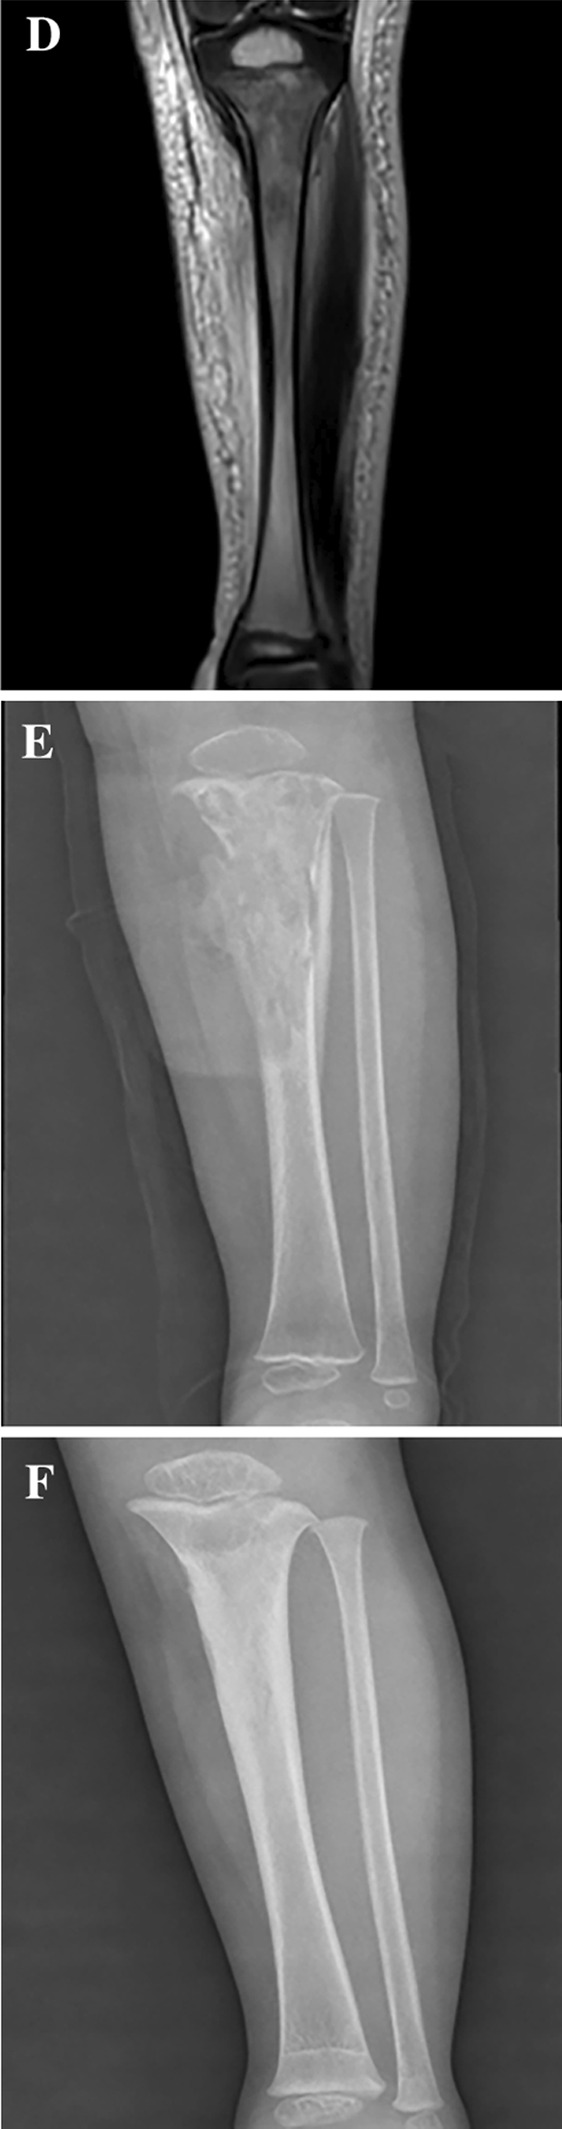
A, B A 2-year-old boy presented with high fever, swelling, and tenderness of the left lower leg. The inflammatory indexes on admission were all elevated. Computed tomography revealed soft tissue swelling of the left lower leg but no fracture or bone destruction. An MRI examination revealed abnormal signals in the proximal tibia soft tissue and bone marrow cavity (C, D). The treatment administered was an antibiotic combination with tibial cortex fenestration, lesion removal, VAC negative pressure drainage; postoperative pathological findings suggested suppurative osteomyelitis. Bacterial culture suggested MRSA, following which the antibiotic was replaced with vancomycin. The VAC negative pressure was removed after four rounds of surgical treatment, and the total duration of hospital stay was 60 days. The inflammatory indicators and body temperature were normal at discharge, and the re-examination radiograph indicated bone destruction at the proximal tibia (E, F). Nine months after surgery, radiographs indicated remodeling of the proximal tibia (G), with normal lower limb function and no postoperative complications
